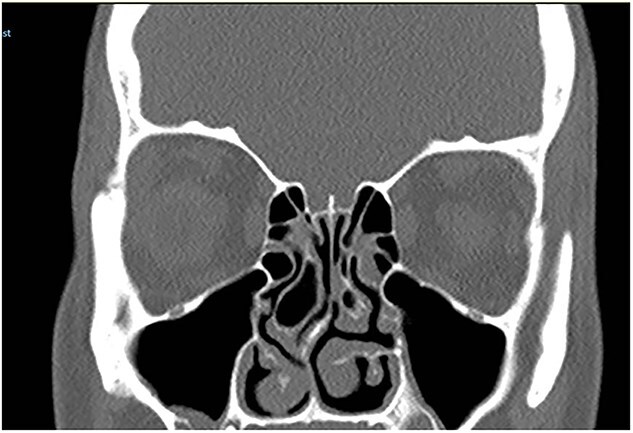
Coronal CT image of the paranasal sinuses demonstrating septal deviation, bilateral inferior turbinate hypertrophy, and inferomedial duplication of the left inferior turbinate.
